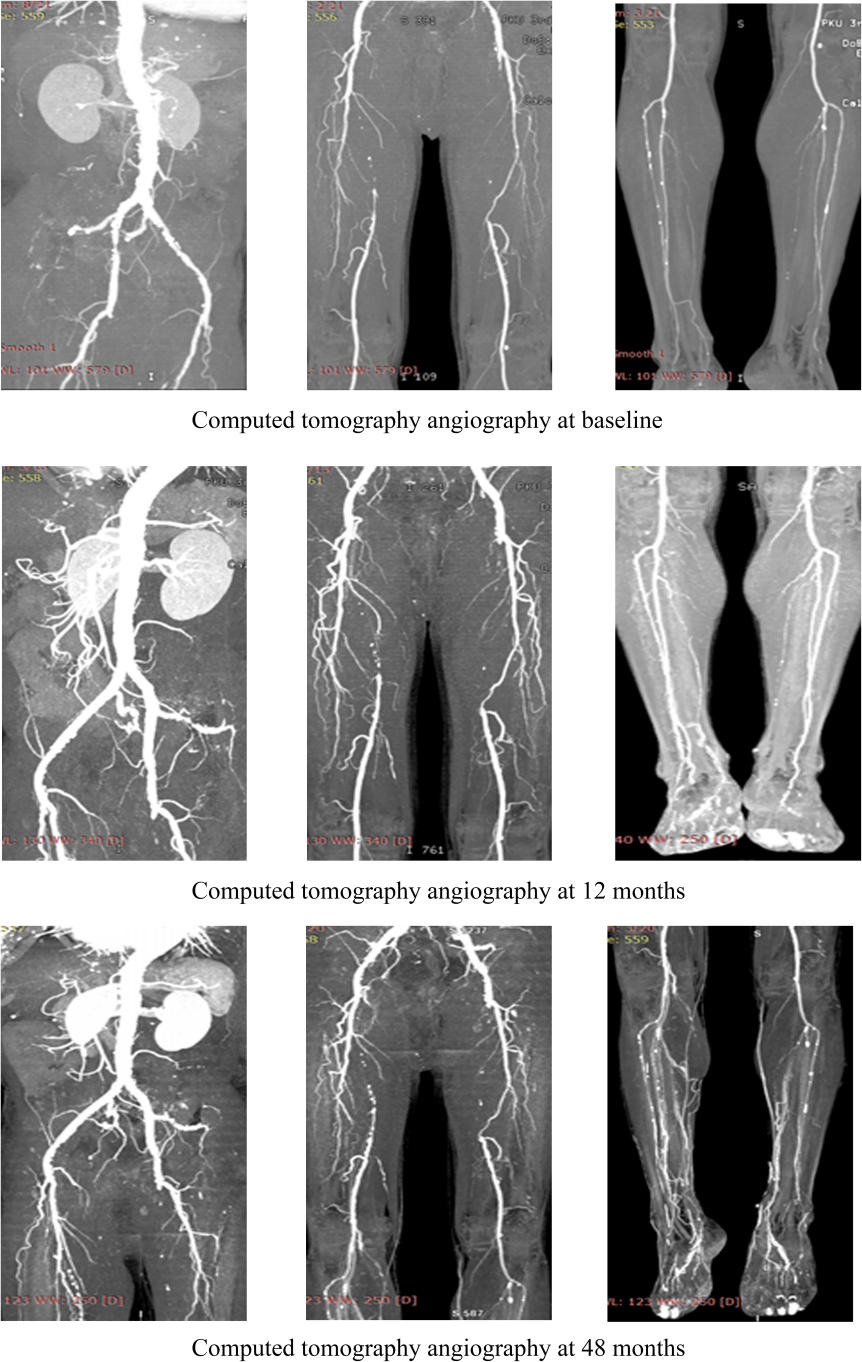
Computed tomography angiography at baseline and follow-up. A 63-year-old male with severe stenosis and occlusive iliac and SFA disease with Rutherford category 3 underwent iliac-only revascularization in December 2015. During follow-up, improvement in the Rutherford categories (3 to 2) and the collateral vessels were observed. The patency of the iliac stent was freedom from significant restenosis or occlusion without any reintervention.
